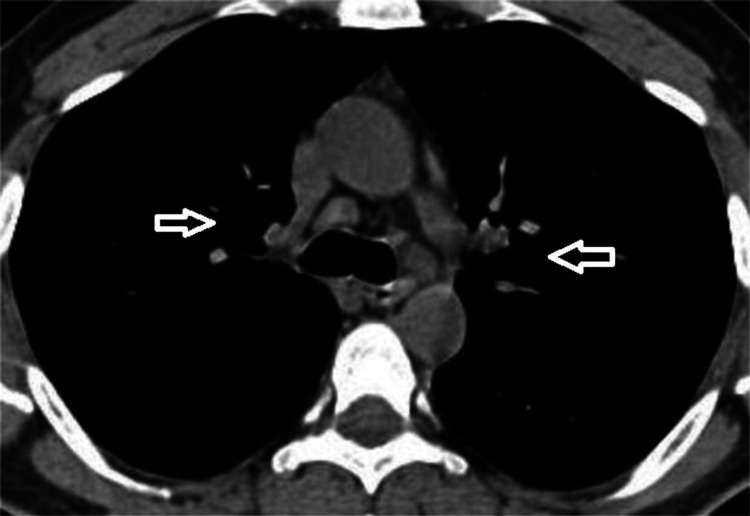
Chest computed tomography at three months. Arrows show the reduction in the diameter of both hilar lymph nodes.
